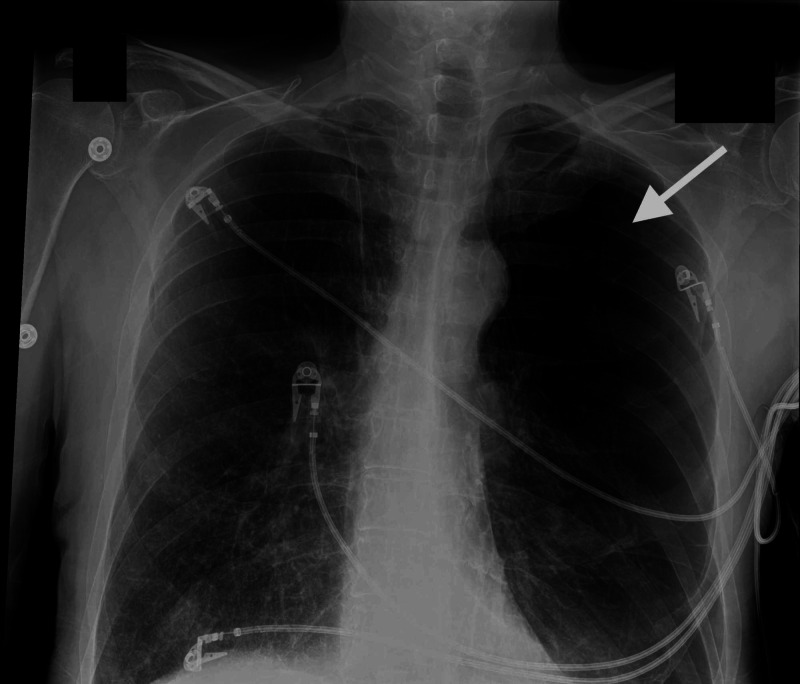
Chest radiograph Chest radiograph (AP view) showing bullous changes involving bilateral upper lobe with severe bullous emphysematous changes particularly in the left hemithorax.
